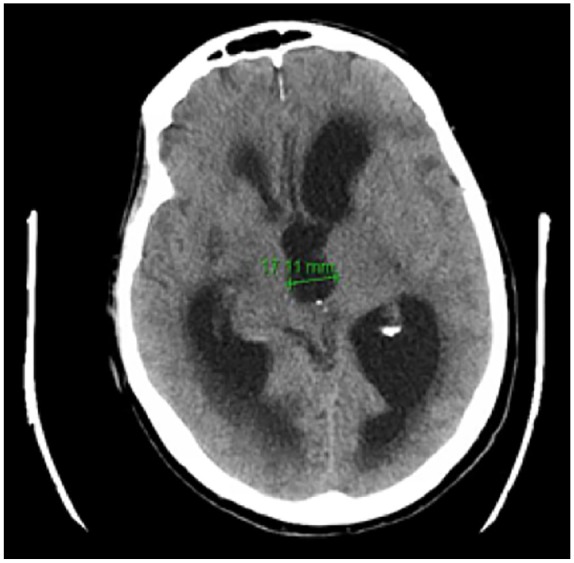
Computed tomography brain scan without contrast revealing internal
